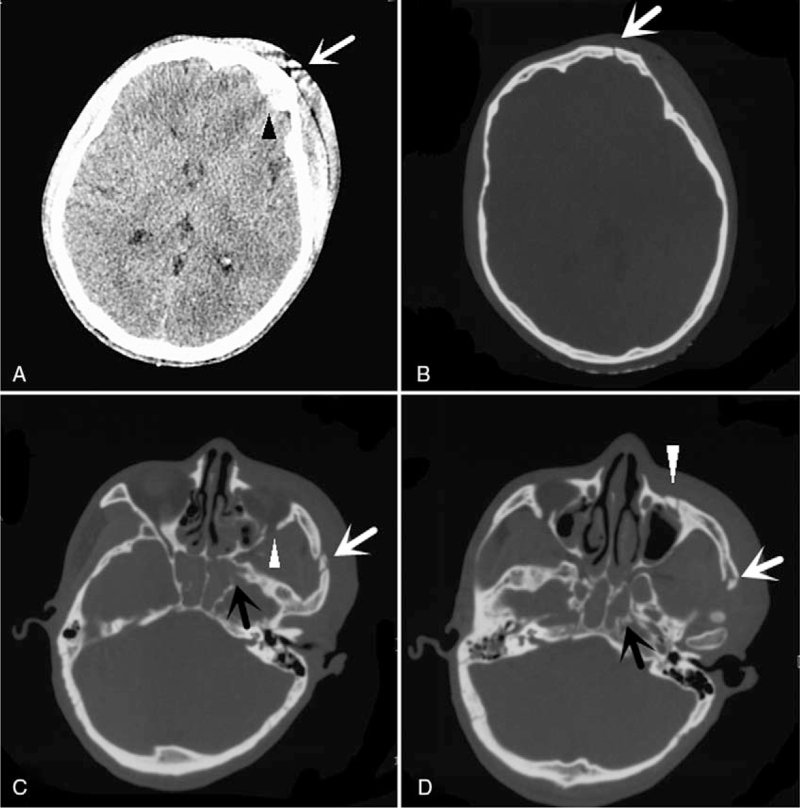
Pre-life CT findings. (A) Left frontotemporal epidural hematoma (black arrowhead) and left frontotemporal top scalp hematoma (white arrow). (B) Left frontal temporal bone fracture (white arrow). (C) Sphenoid sinus fracture and blood accumulation in sphenoid sinus (black arrow), left zygomatic arch fracture (white arrow), and fracture of lateral orbital wall (white arrowhead). (D) Sphenoid sinus fracture and blood accumulation in sphenoid sinus (black arrow), left zygomatic arch fracture (white arrow), and left anterior wall of maxillary sinus fracture (white arrowhead).
